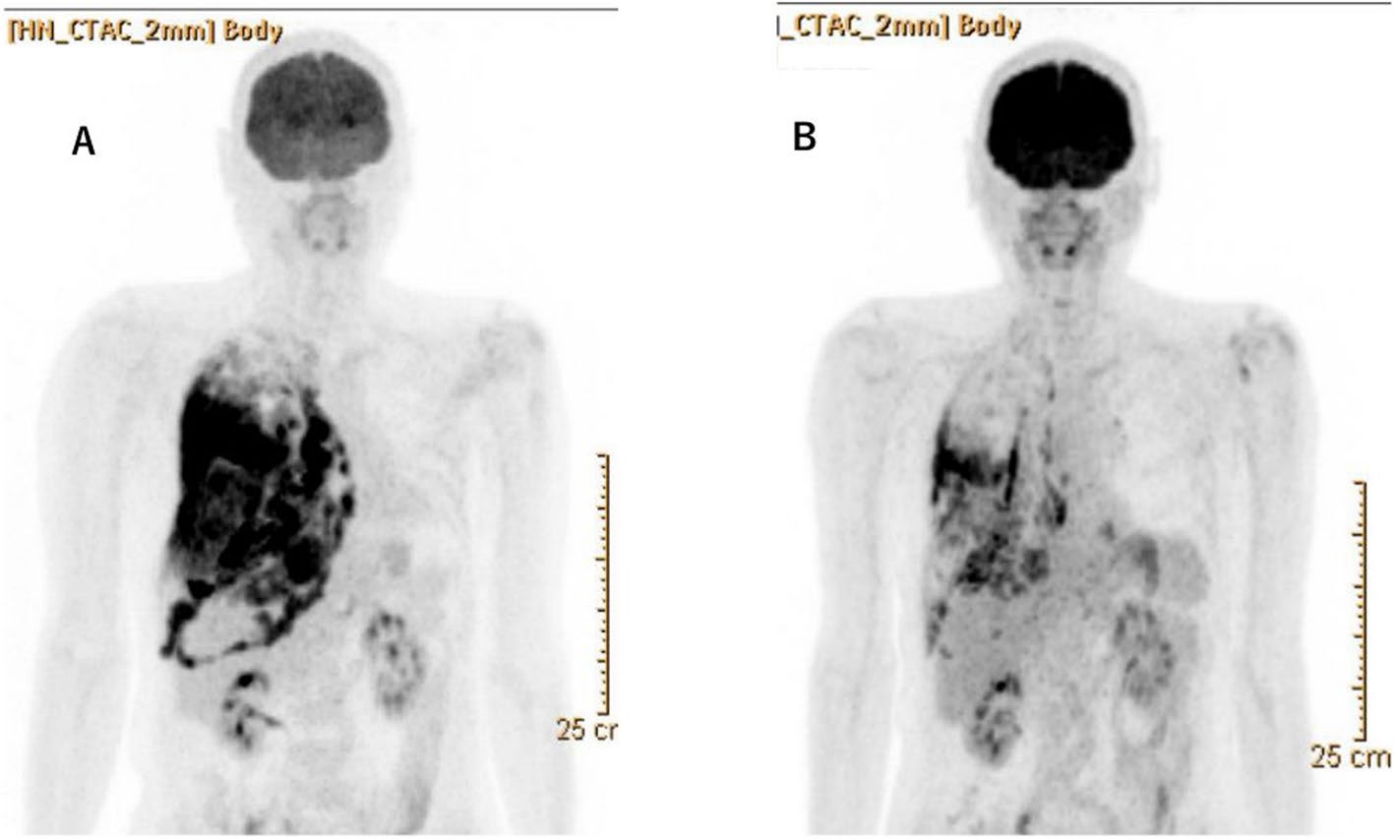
Findings of F-18 fluorodeoxyglucose (FDG) positron emission tomography (PET). A Before chemotherapy, FDG uptake was remarkable in the entire parietal pleura. B After 2 cycles of chemotherapy, FDG uptake was decreased and disappeared in some areas
